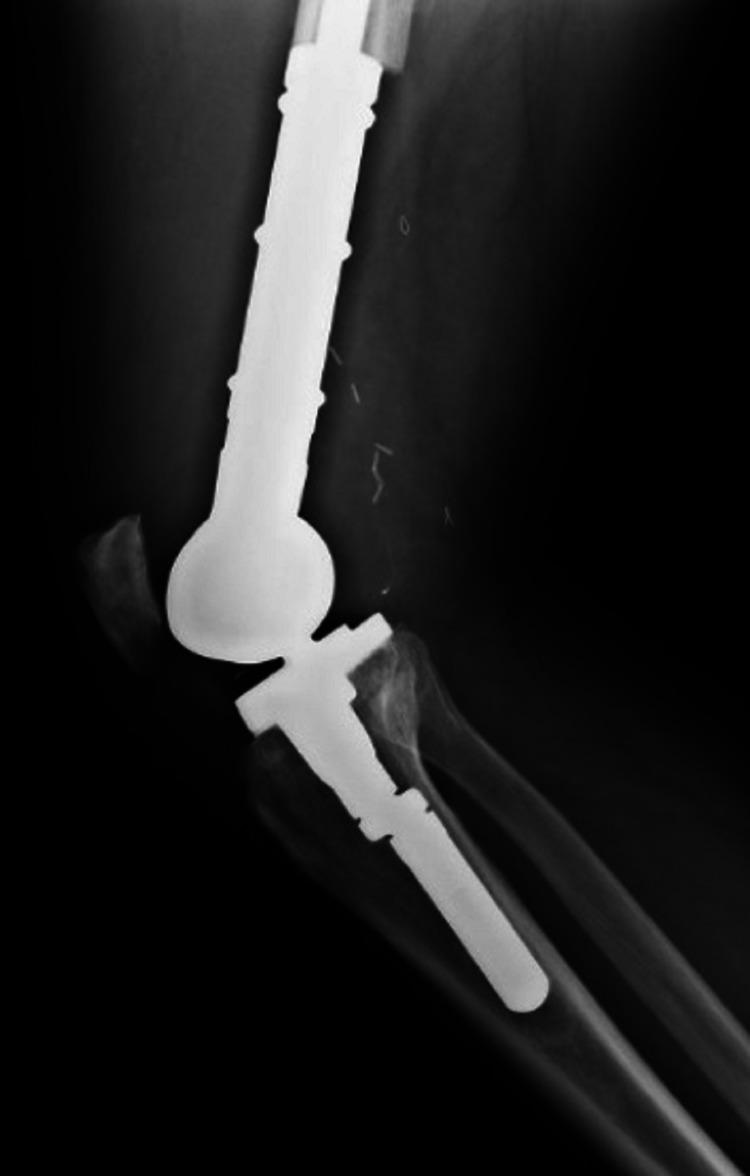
Lateral right knee radiography Knee replacement with prosthetic material
